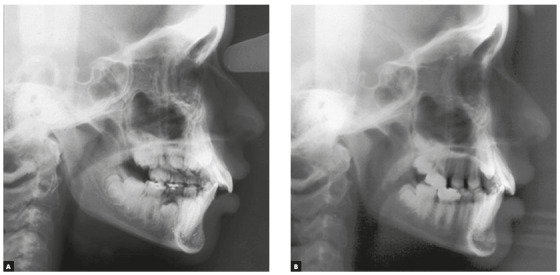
A) Initial radiograph, at the beginning of the treatment. B) Radiograph obtained after cervical headgear treatment.
